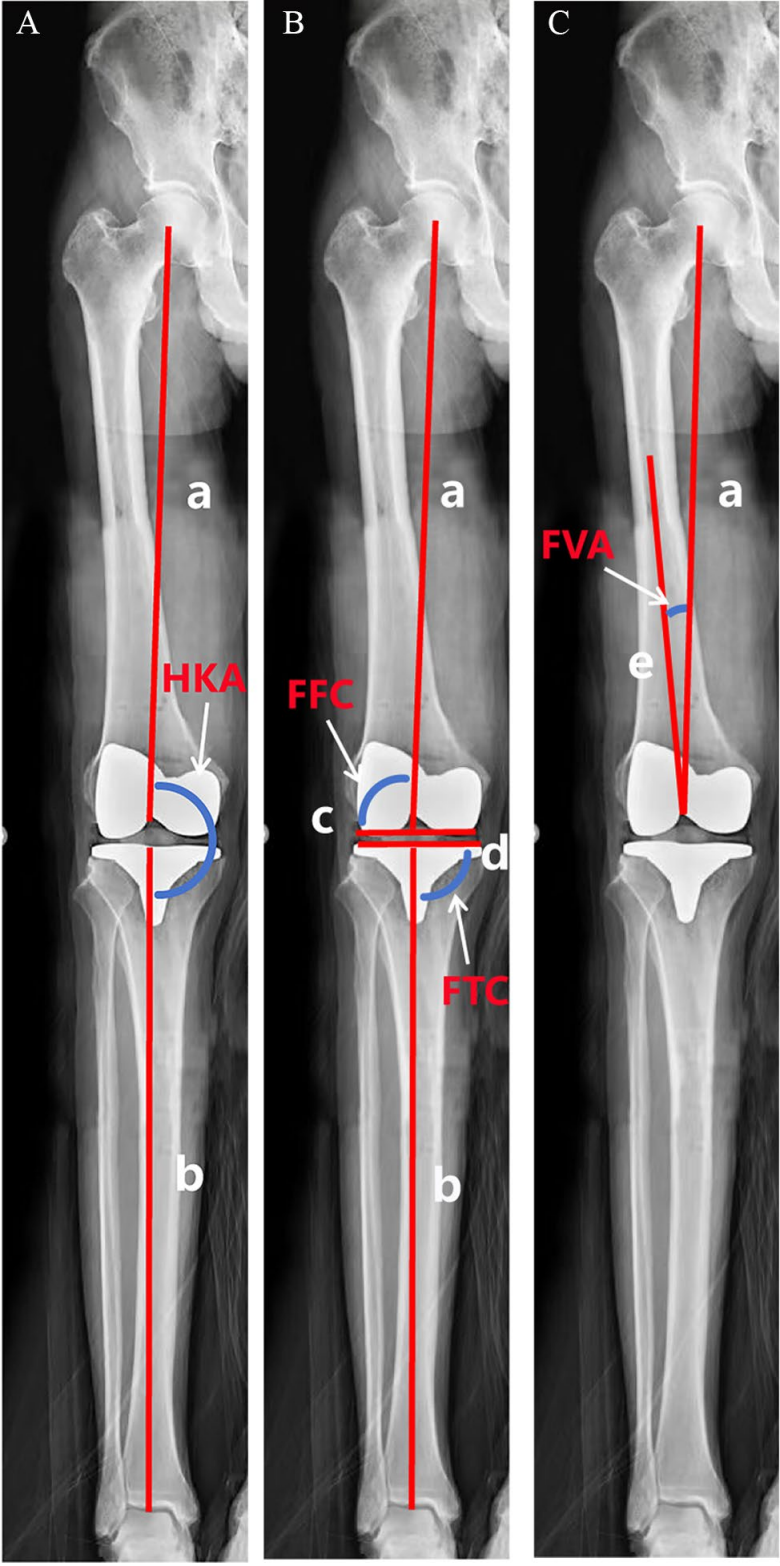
Measurement of HKA, FFC, FTC, FVA. (A) Line a was the femoral mechanical axis, and line b was the tibial mechanical axis; the medial angle formed between them was recorded as the HKA. (B) Line c was the line across the bottom of the femoral condyles, line d was the line across the bottom of the tibial plateau on the anteroposterior radiograph; the lateral angle between line a and line c was recorded as FFC, and the medial angle between line b and line d was recorded as FTC. (C) Line e was the anatomical axis of the femur, the acute angle between line a and line e was recorded as FVC.
